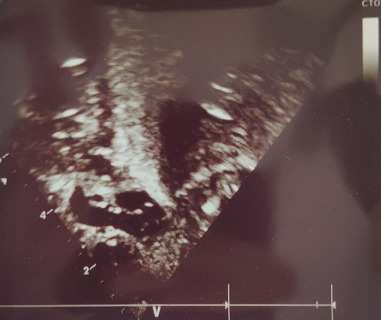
Echocardiography of a 3-day-old neonate with ectopia cordis, showing no major cardiac defects.
